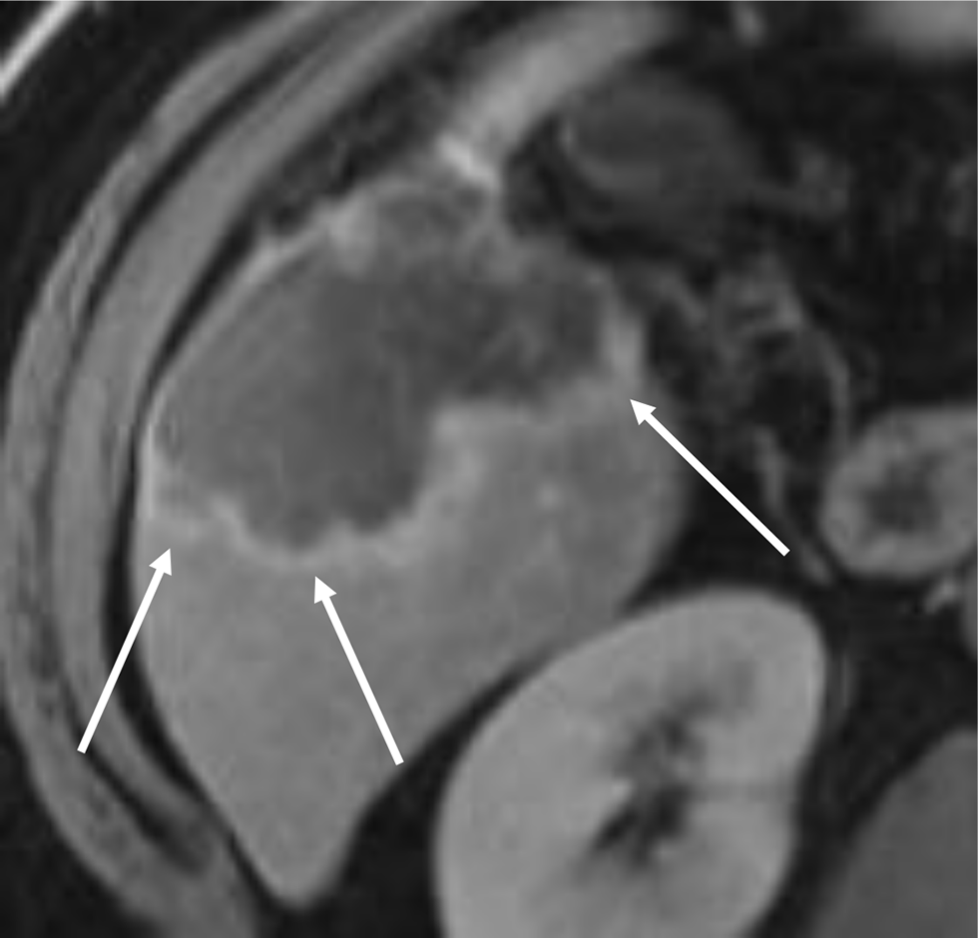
Lobulated Margins. Axial T1-weighted MRI in an early delayed phase demonstrates a peripheral mass forming CCA in the right hepatic lobe with lobulated margins (arrows) and rim enhancement
